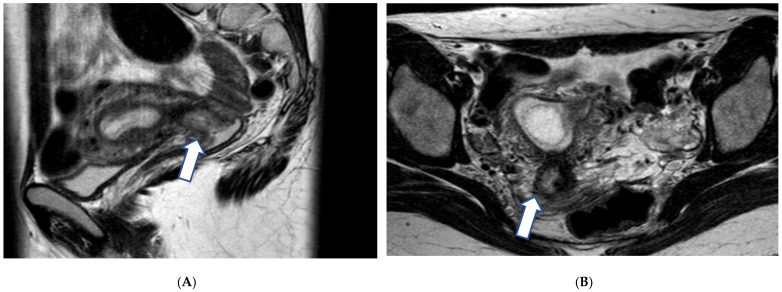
A 29-year-old gravid at gestational week 7, with biopsy confirming grade II squamous cell cervical cancer. T2-weighted images in the sagittal (A) and axial (B) plane demonstrate an ill-defined intermediate signal intensity mass within the cervical canal disrupting the low signal intensity stromal rim on the right (arrows).
